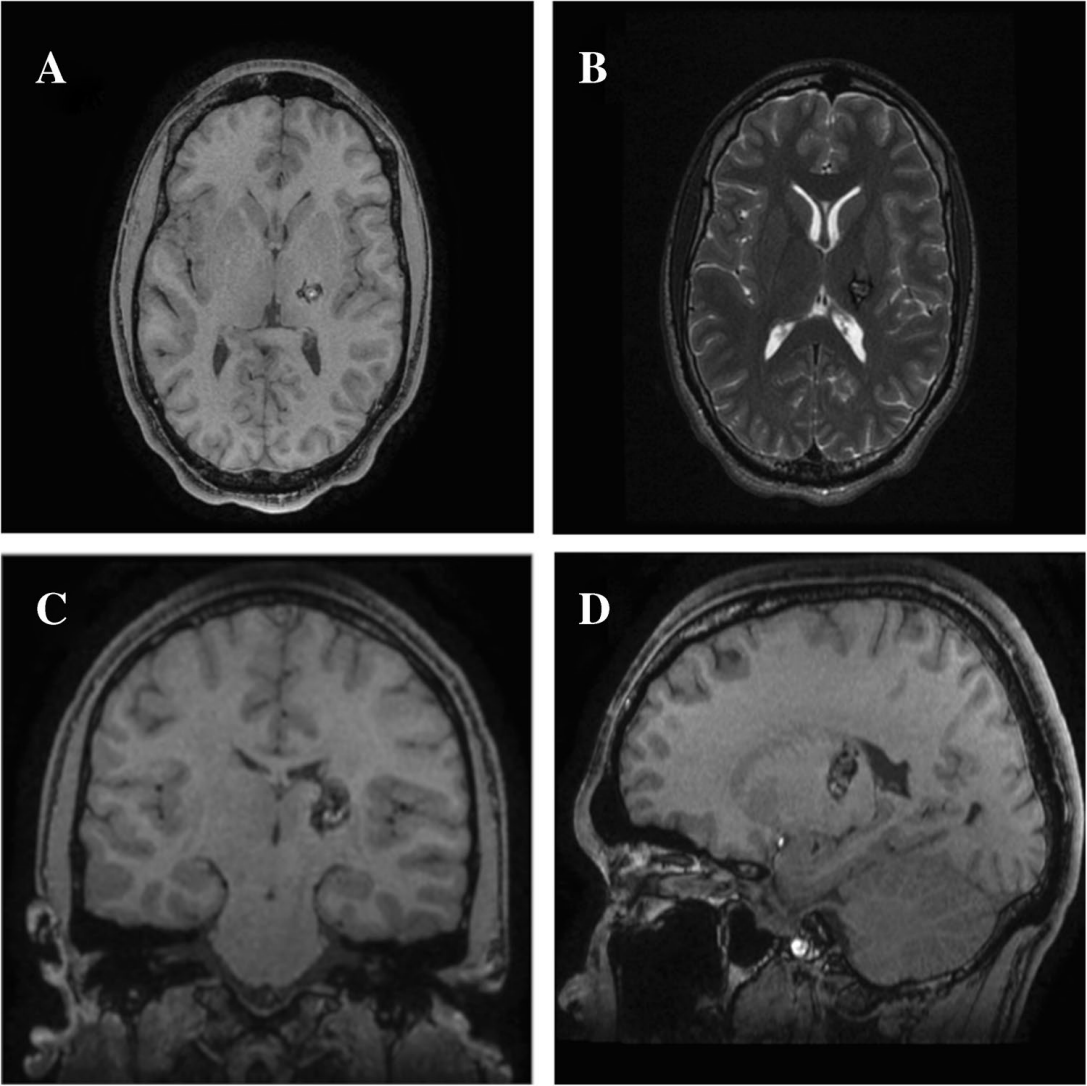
Preoperative non-contrast magnetic resonance imaging showing a hyperintense lesion within a hypointense haemosiderin ring in the left lateral thalamus, consistent with a cavernoma (A axial T1-weighted; B axial T2 weighted; C coronal reconstruction of T2 weighted: and D sagittal reconstruction of T1-weighted images)
